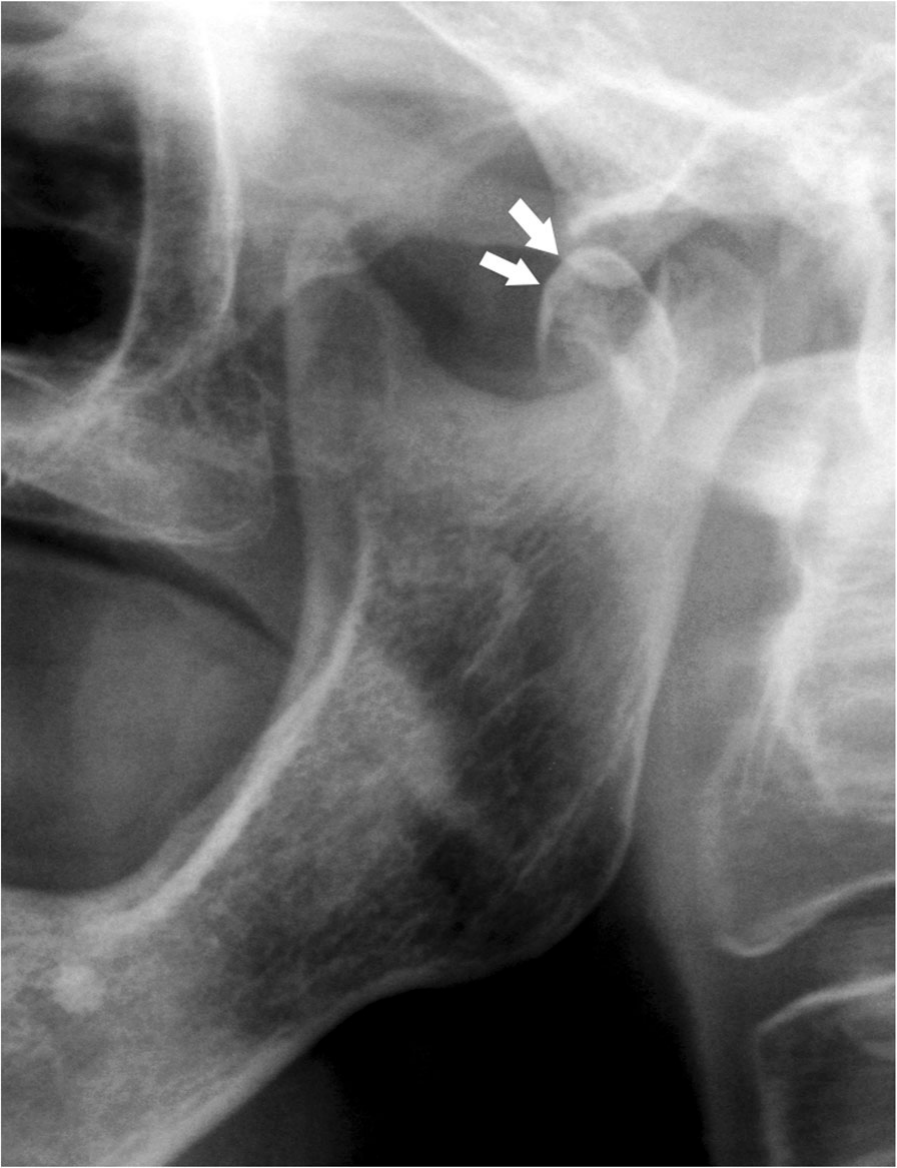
Condylar process fracture. Dislocation. Cropped panoramic radiograph. The bone fragments are not in line and the condylar head moves out from the glenoid fossa (arrows)
